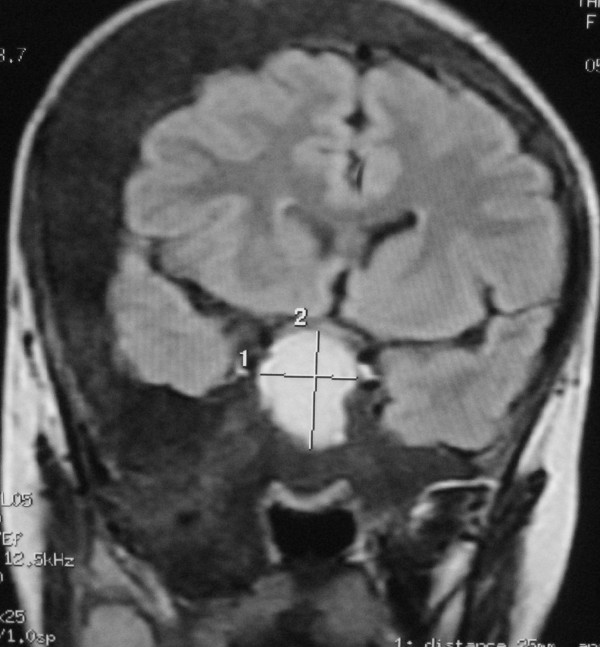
T1-weighted sagittal MRI scans of the head. Optic chiasma is compressed.
